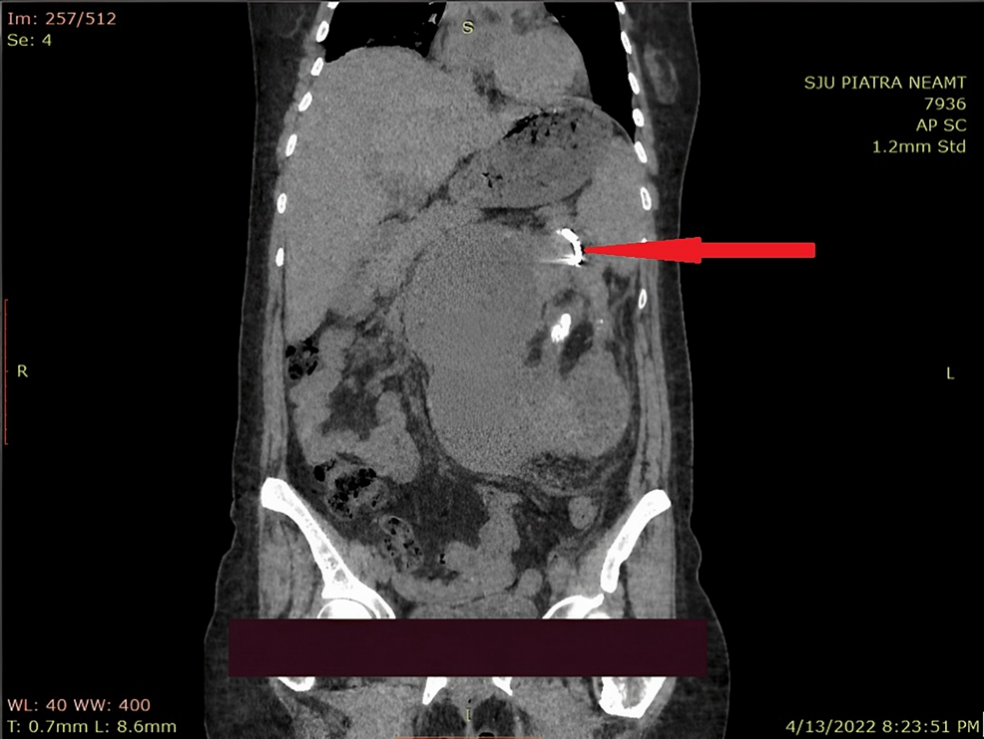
CT scan showing the stent in the collecting system but with the incomplete evacuation of the infected urine
